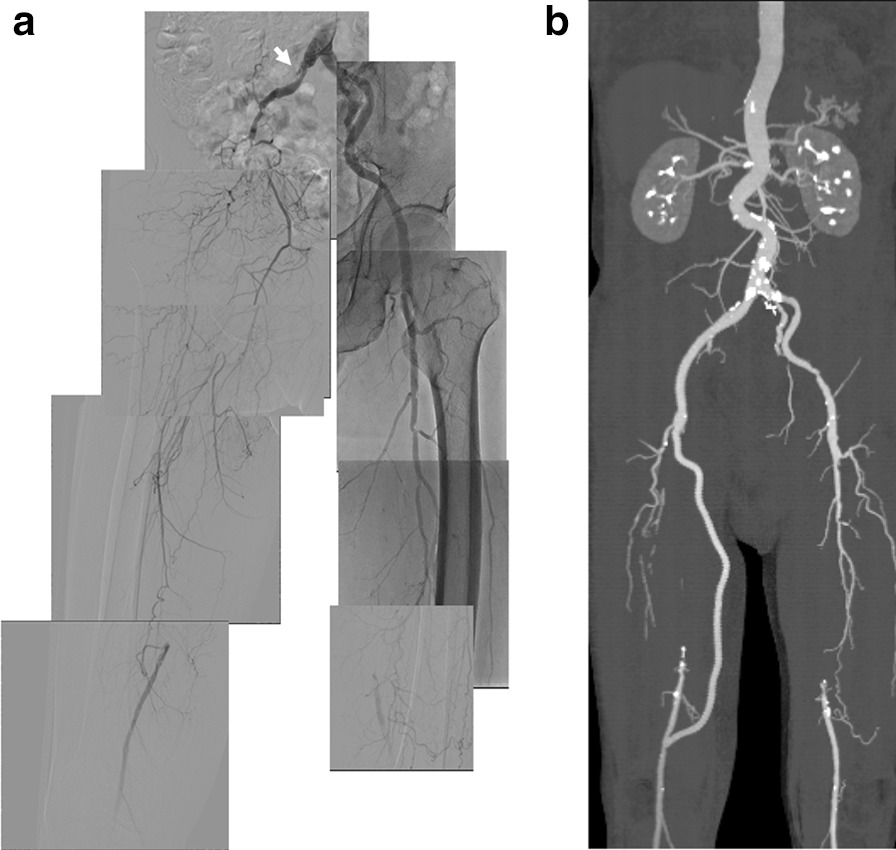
Lower extremity angiography and multislice computed tomography (MSCT) at the first hospitalization. a Lower extremity angiography in January 2019. The right external iliac artery was occluded (white arrow), and the right superficial and deep femoral arteries were also occluded. The right femoral artery was mainly maintained by collateral flow from the right internal iliac artery. b After aortofemoral and femoropopliteal bypass grafts
